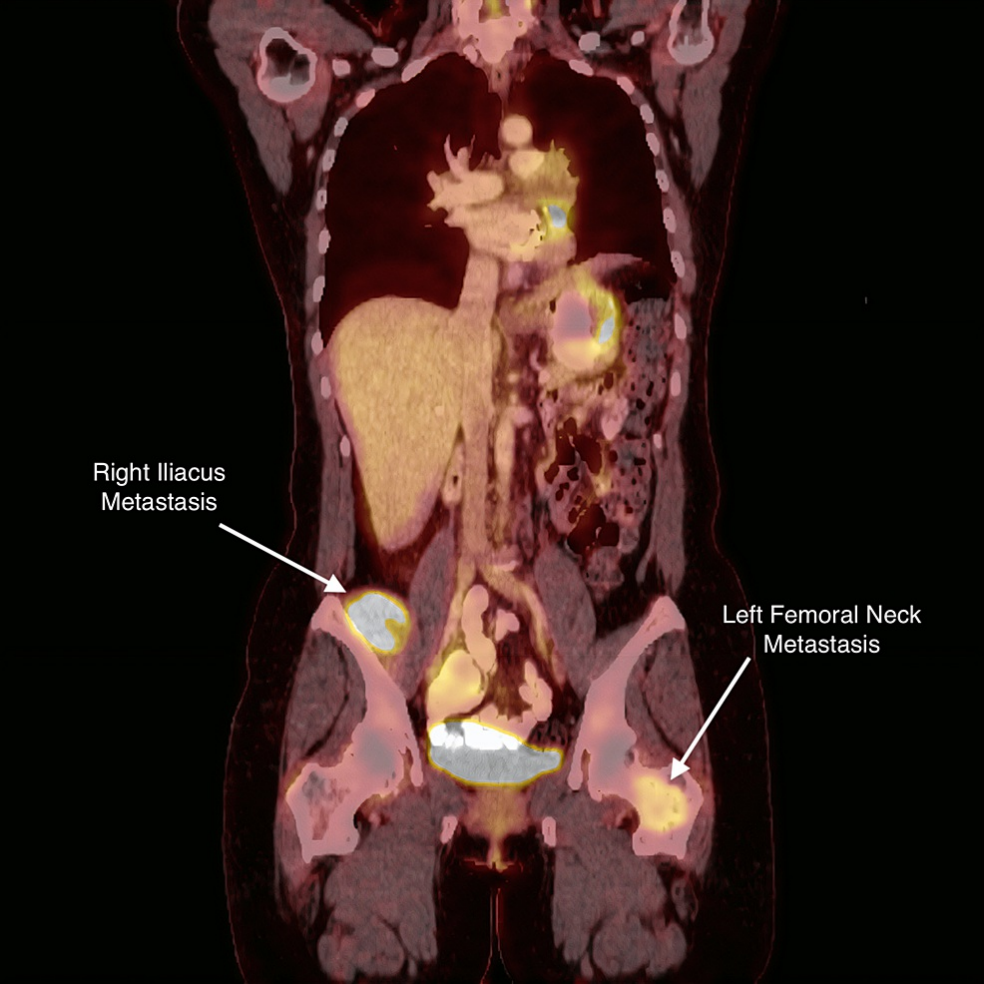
Repeat PET CT scan, conducted on 1/13/2020, demonstrating multiple new hypermetabolic osseous lesions involving the iliacus muscle and femoral neck. No demonstrable evidence of local recurrence within the heart.
